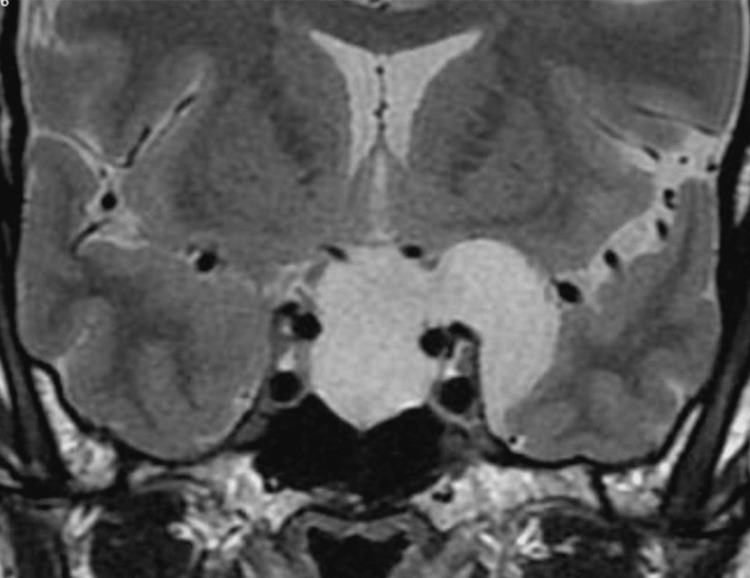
Coronal T2- weighted magnetic resonance imaging of the sellar region, showing hyperintense cavity within the sella, extending into the left middle fossa through the superior part of the cavernous sinus
